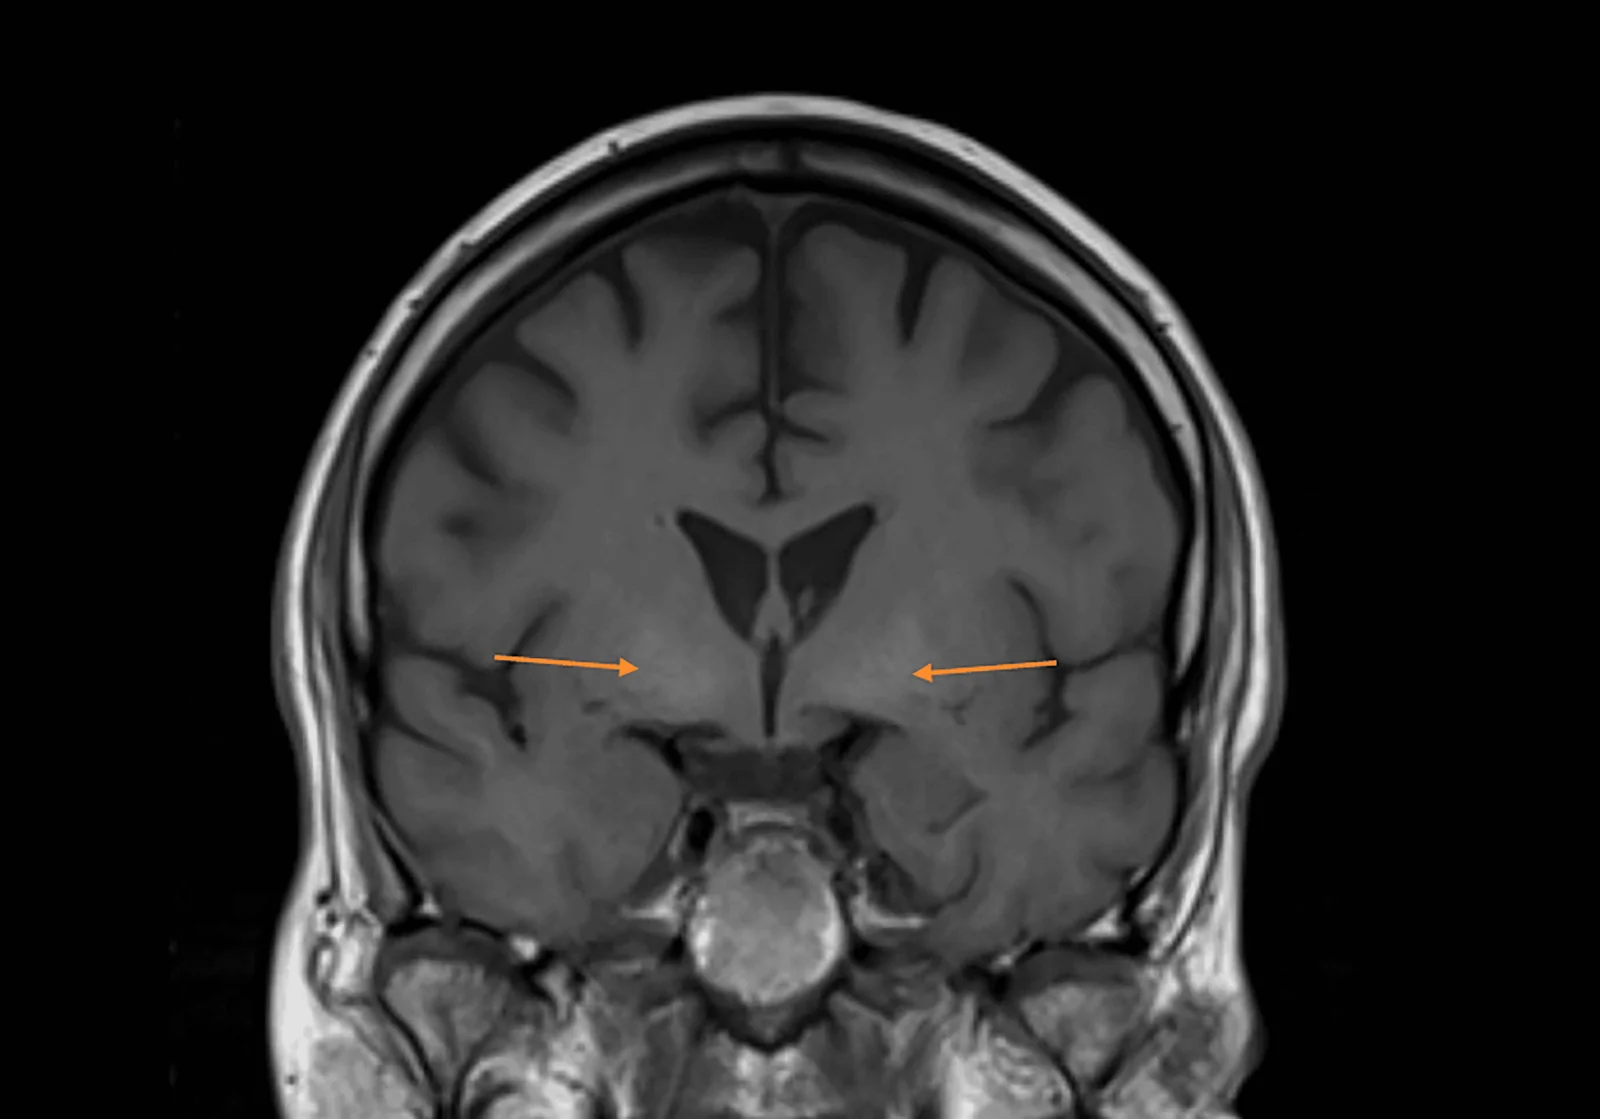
MRI Brain showing symmetrical bilateral hyper-intensities in globus pallidus (marked by orange arrow).
